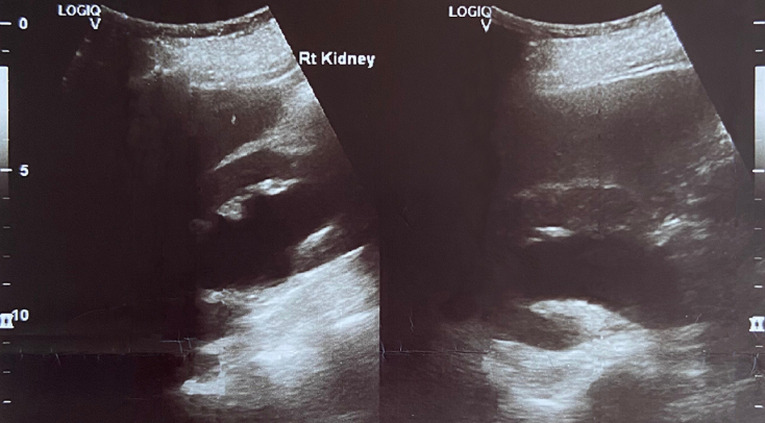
preoperative ultrasound imaging of the left kidney
